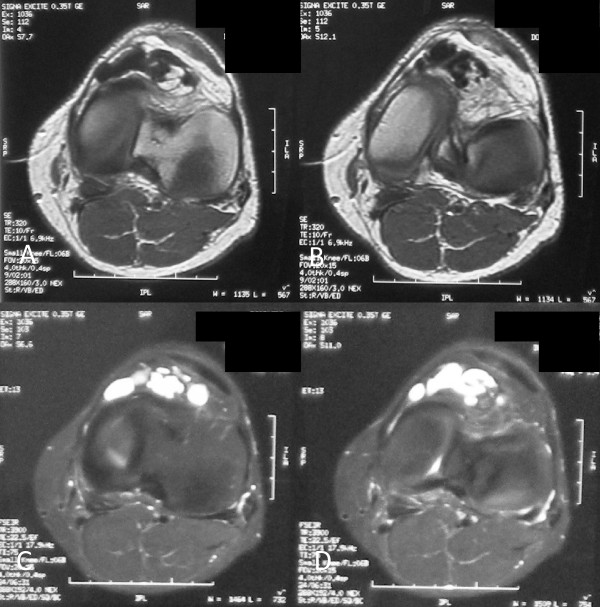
Axial magnetic resonance images on the level of the inferior patellar pole. (A, B) Sequential T1-weighted images. (C, D) Sequential T2-weighted images.
